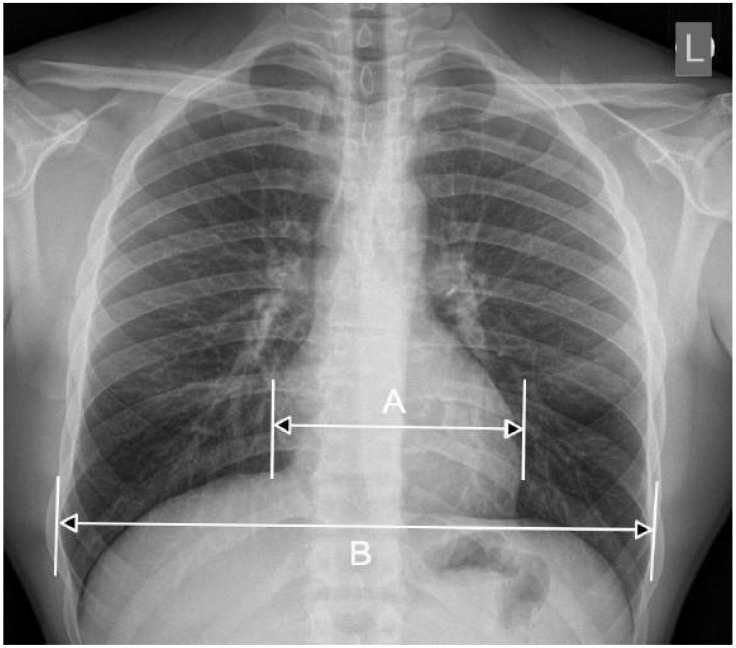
The approach to calculating the CTR involves measuring the relationship between the transverse diameter of the heart (denoted as [A]) and the transverse diameter of the chest cavity (denoted as [B]), as observed on a chest radiograph taken in the posteroanterior (PA) view. The CTR is then computed using the following formula: CTR = A/B [7].
